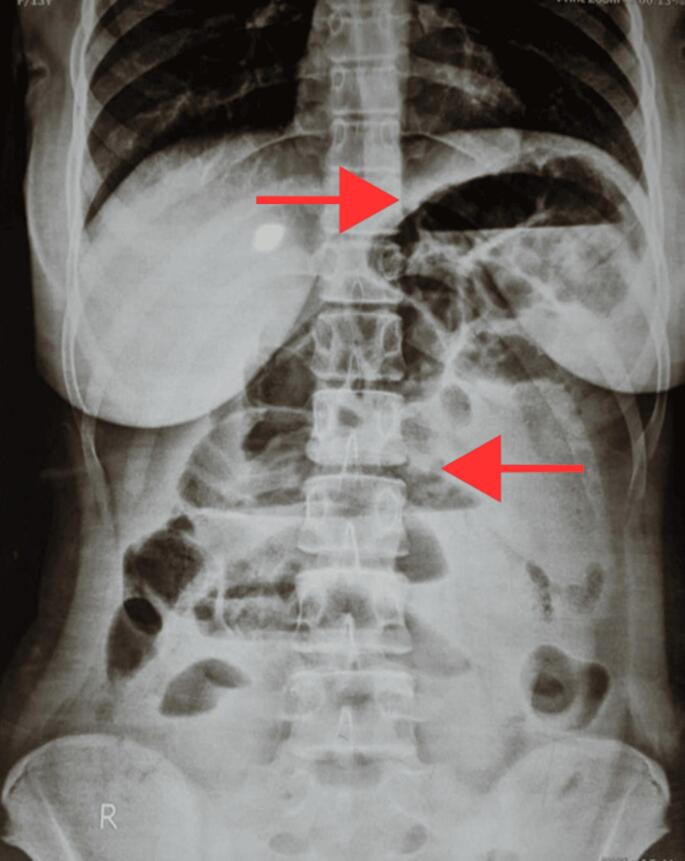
Abdominal X-ray showing central multiple air-fluid levels.
